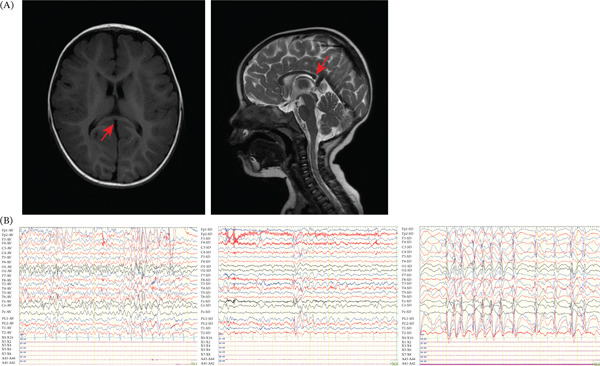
Brain MRI and EEG in our patient. (A) Brain magnetic resonance imaging in the patient. Brain MRI demonstrated thinning of the splenium of the corpus callosum at the age of 1 year (left), whereas follow‐up MRI showed a normal appearance of the splenium at the age of 2 years (right). (B) EEG in the patient. During the awake state, bilateral occipital regions show an 8–9‐Hz low‐to‐medium amplitude alpha rhythm, intermixed with a small amount of low‐amplitude fast activity. The rhythm is approximately symmetrical between hemispheres, with poor amplitude modulation (left); EEG during the waking period shows frequent generalized 1.5–2.5 Hz high to extremely high amplitude sharp and slow wave complexes, sometimes with left‐sided predominance; during the sleeping period, the EEG demonstrates generalized paroxysms of 1.5–2.5 Hz high to extremely high amplitude sharp and slow wave complexes. Focal epileptiform discharges with left‐sided predominance.
